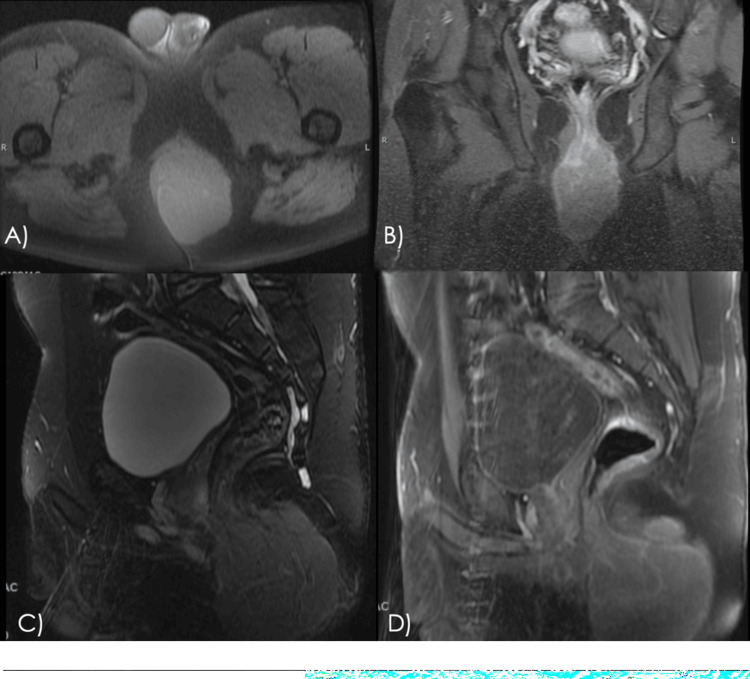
Baseline contrast-enhanced MRI Pelvic contrast-enhanced MRI. T1-weighted axial (A) and coronal post-gadolinium T1-weighted (B) images, both with fat saturation. The coronal image shows tumoral infiltration of the internal anal sphincter. T2-weighted sagittal (C) and post-gadolinium T1-weighted sagittal (D) images, both with fat saturation. A lobulated mass involves the anal canal and shows heterogeneous enhancement. MRI, magnetic resonance imaging
